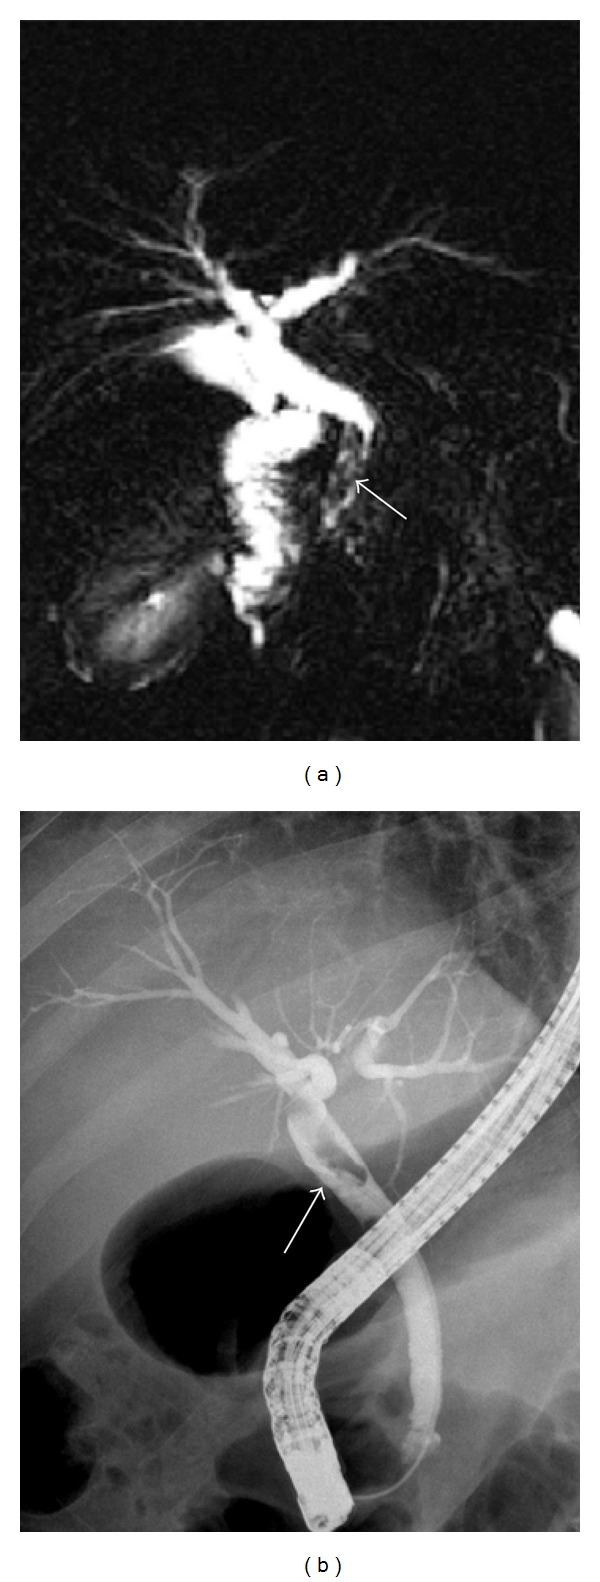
MRCP image demonstrates an irregular heterogenous hypointense region in the distal common bile duct (arrow). On MRI, this region evaluated as benign pathology such as calculus and sludge. ERCP image demonstrates a filling defect in this region (arrow). This filling defect was determined as Fasciola hepatica and extracted during the procedure.
